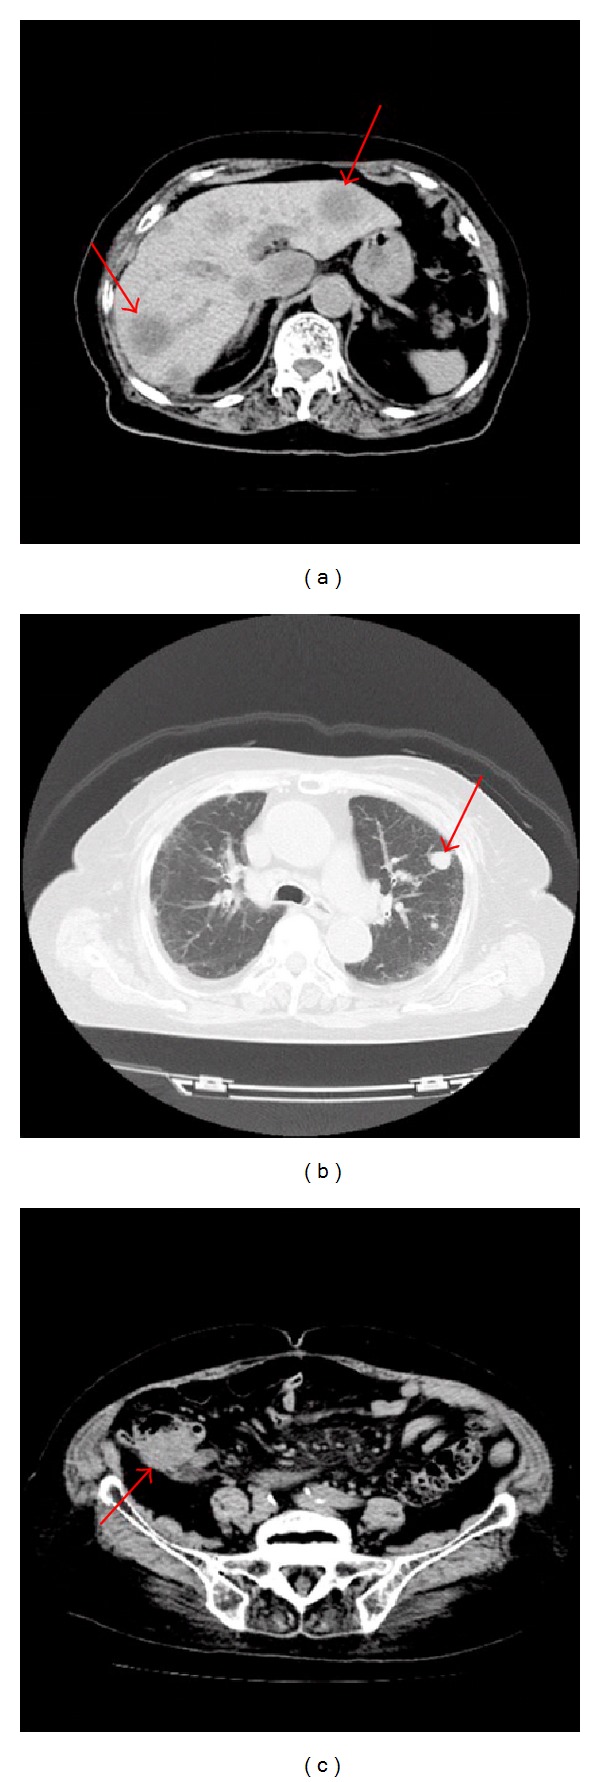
Images of systemic CT examination from the liver (a), the lung (b), and the abdomen (c). The arrow surrounding the appendix in the panel (c) may indicate the primary site of the cancer. The arrows in (a) show multiple metastasis of the liver. The arrow in (b) indicates the metastasis of the lung.
